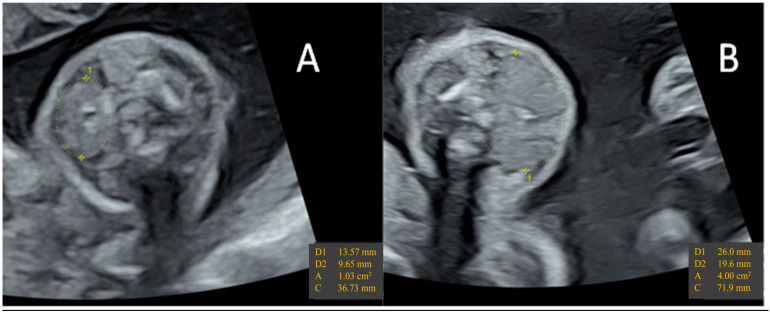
Sonographic views of fetal thyroids in an axial plane at 23 WG (personal data from Bicetre Hospital Fetal Medicine Unit). Image (A) represents a normal fetal thyroid with a transverse diameter of 13.57 mm and a perimeter of 36.73 mm, whereas image (B) represents a large hypothyroidic fetal goiter with a transverse diameter of 26.0 mm and a perimeter of 71.9 mm.
